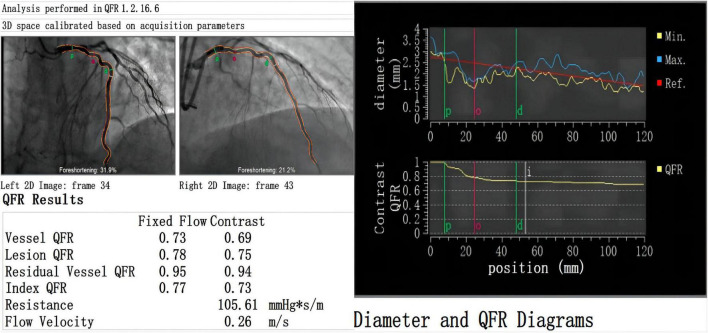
Case example of QFR report. There is a moderate stenosis (diameter stenosis = 46.4%) with QFR value = 0.69 in a 58-year old male patient. Qm (33 ml/min) and PI (3), the backflow component of TTFM is not evident. Postoperative multislice computed tomography angiography shows patency of the LITA graft.
